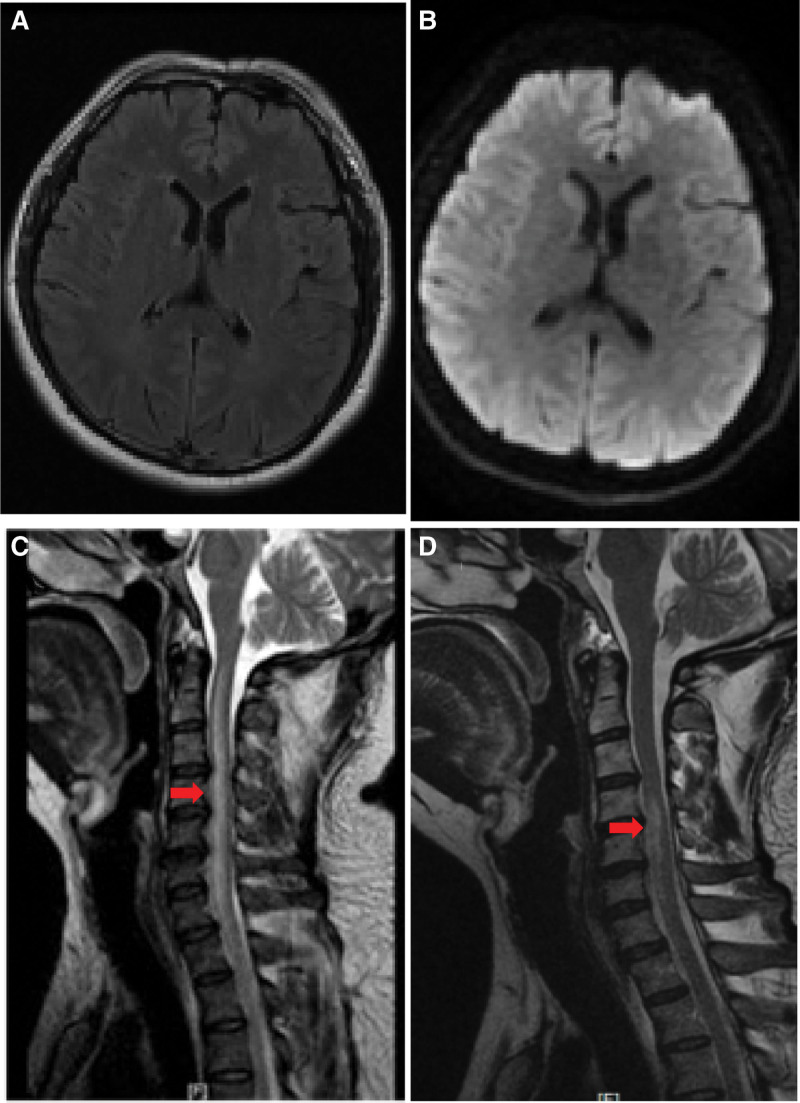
Brain, cervical, and thoracic MRI. (A) Brain FLAIR imaging 3 hours post-procedure showed no abnormalities. (B) No anomalies were detected in the DWI. (C) A lesion with elevated T2 signal intensity was identified at the anterior cervical spinal cord between C1 and T1 on hospital day 4. (D) MRI of the spinal cord on hospital week 3 demonstrated a decrease in the hyperintense region. DWI = diffusion weighted imaging, MRI = magnetic resonance imaging.
